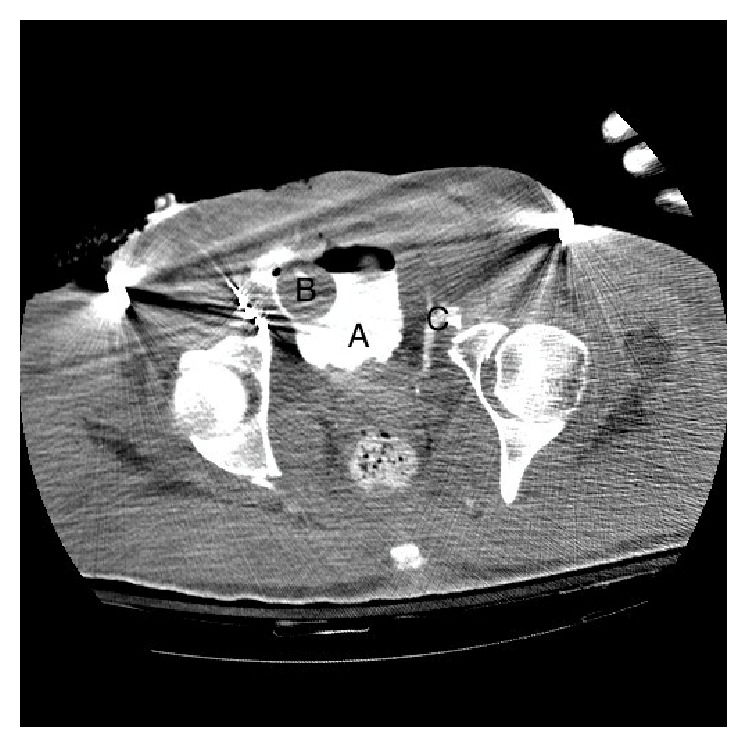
CT A/P demonstrating extent of injuries noted on presentation. A: bladder with contrast. B: balloon of suprapubic tube. C: contrast extravasation outside of the bladder.
